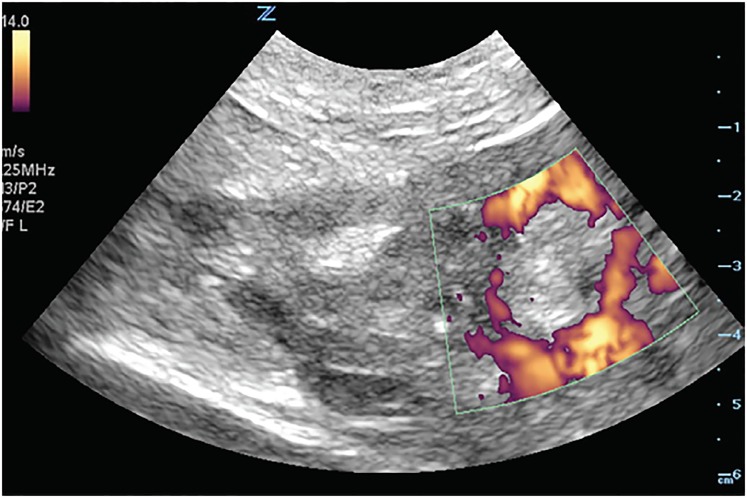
Transabdominal power Doppler ultrasound of the left adnexa. Note ectopic pregnancy with “ring of fire” sign reflecting peripheral hypervascularity.
